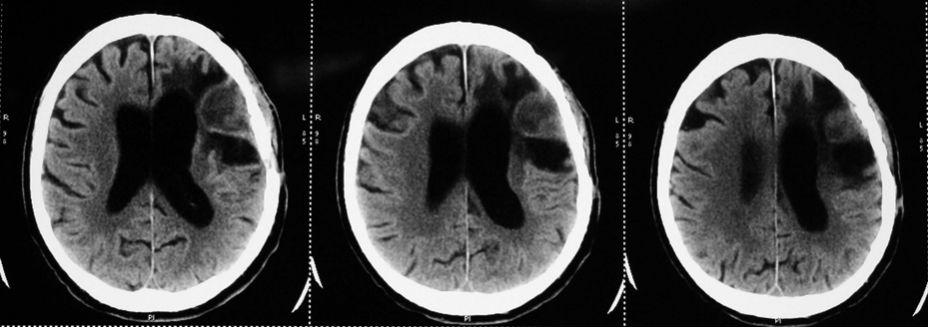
Preoperative CT scan. The surrounding tissues (including the dura) are thicker, related to the associated inflammation.
